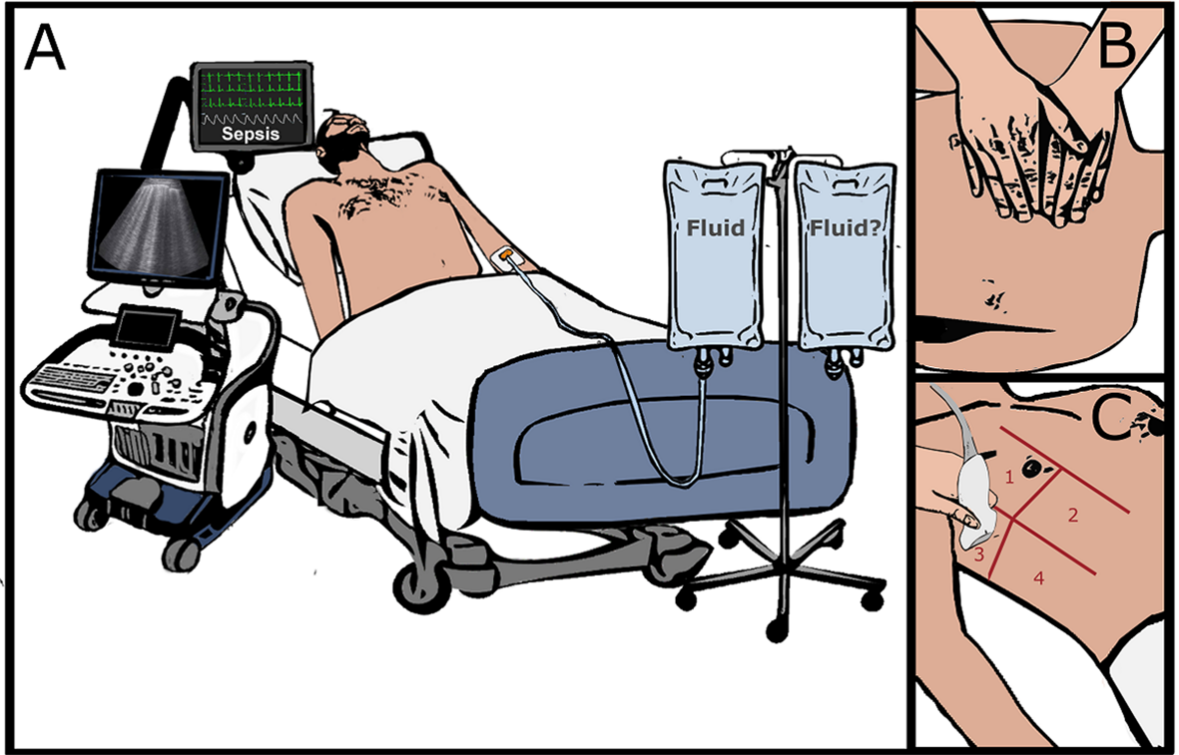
Bedside Lung Ultrasound in Emergency (BLUE) protocol.
